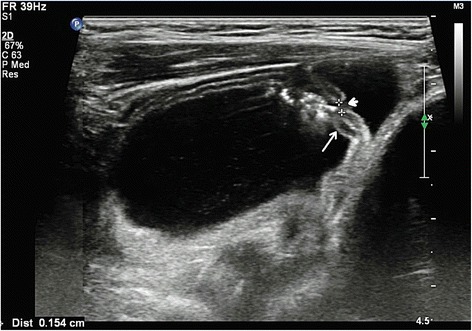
Ultrasonography showing dilatation of the duodenum and proximal jejunum. A membrane (*arrow*) and a pinhole (*small arrowhead*) are seen in the jejunal membrane
